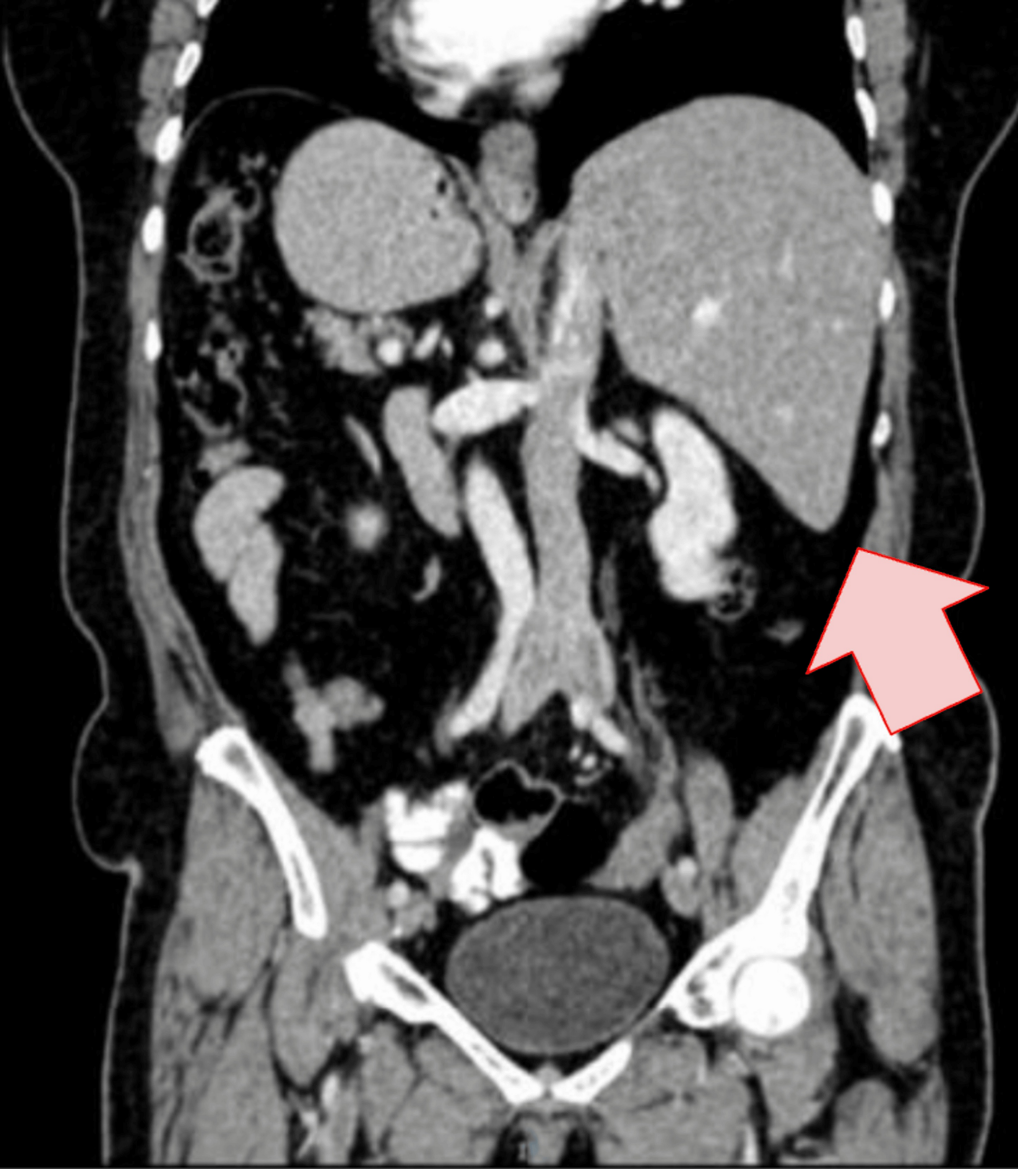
Contrast-enhanced abdominopelvic computed tomography demonstrating situs inversus totalis Coronal contrast-enhanced abdominopelvic computed tomography demonstrating mirror-image transposition of the abdominal viscera, with the liver located in the left upper quadrant (arrow), consistent with situs inversus totalis.
